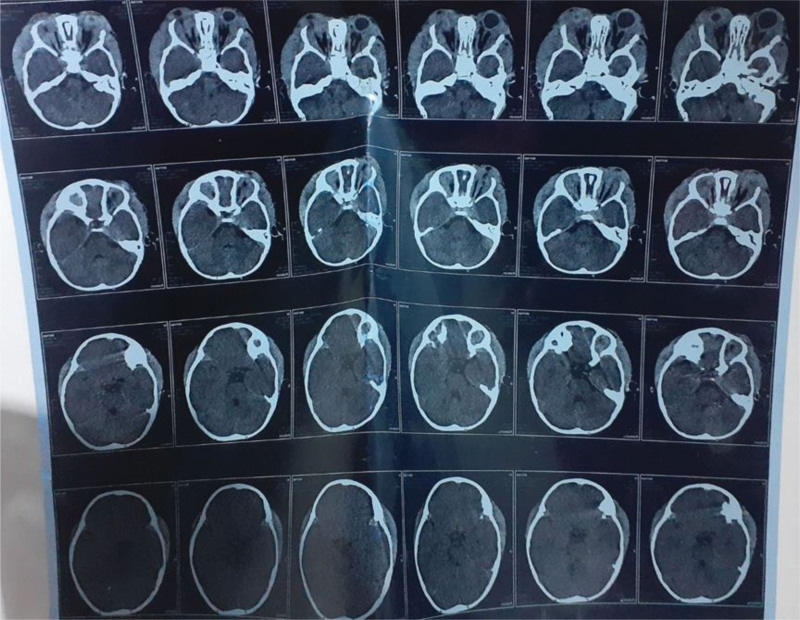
CT scan of the head and orbit revealed anterior displacement of the right eye globe without bone erosion. CT = computed tomography.
